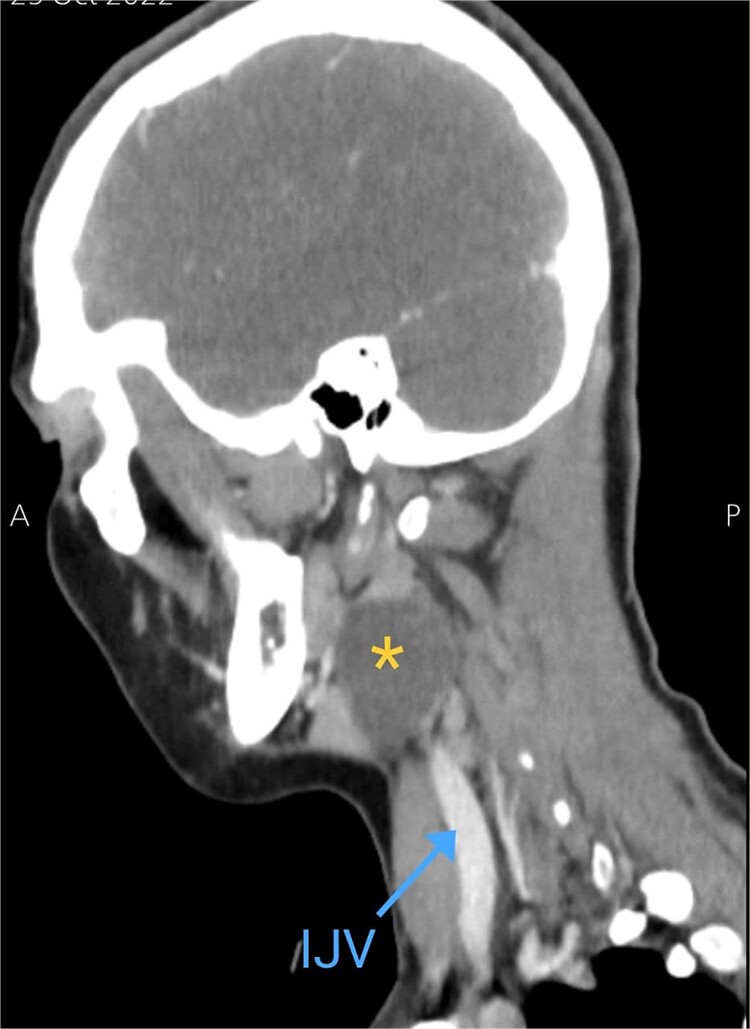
Sagittal CT neck scan showing the branchial cleft cyst. Asterisk: branchial cleft cyst, blue arrow: IJV.
